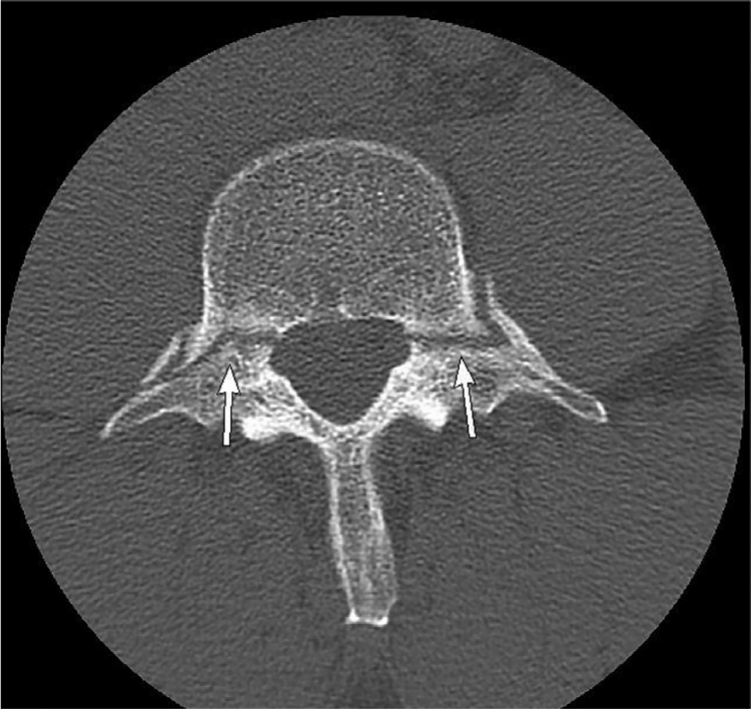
Axial CT showing bilateral transverse fracture of L4 pedicle.
